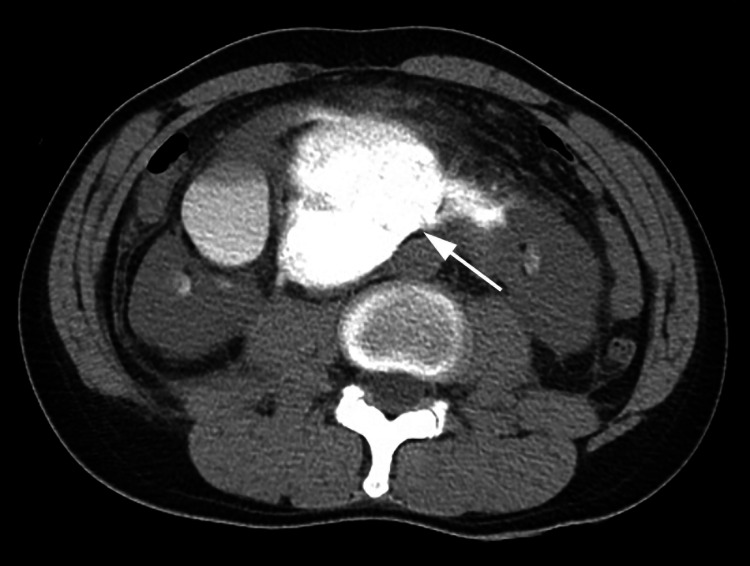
Plain abdominal CT on day 2 revealed contrast accumulation in the retroperitoneum (arrow).
